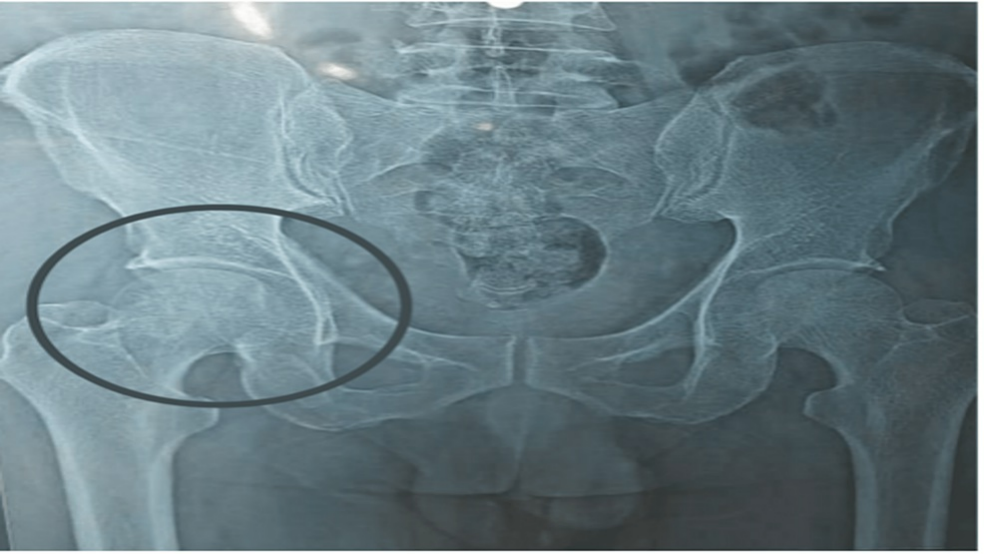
Anteroposterior pelvic radiograph showing sclerosis or cystic abnormalities in the femoral head. The joint space was protected.
